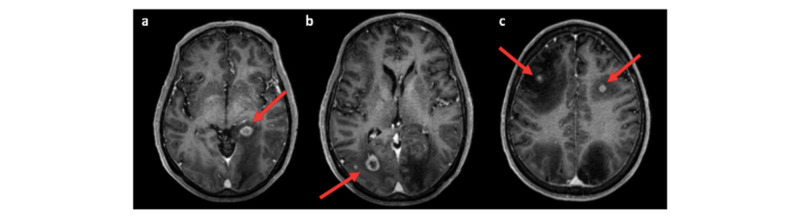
Axial T1 MRI of brain Axial post-contrast T1 MR images of the brain demonstrating ring-enhancing lesions (red arrows) in the (a) left occipitotemporal, (b) right occipital, and (c) bifrontal regions with significant surrounding vasogenic edema.
